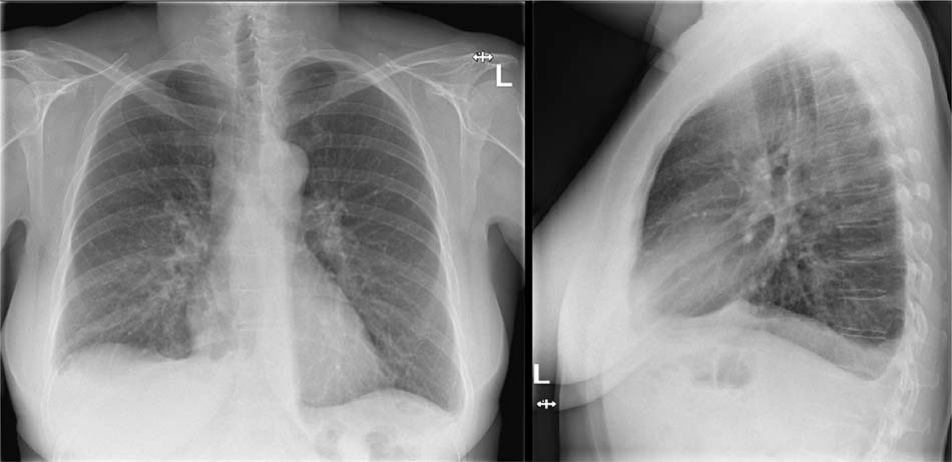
Chest X-ray during visit to the emergency department showing bilateral pleural effusion.
